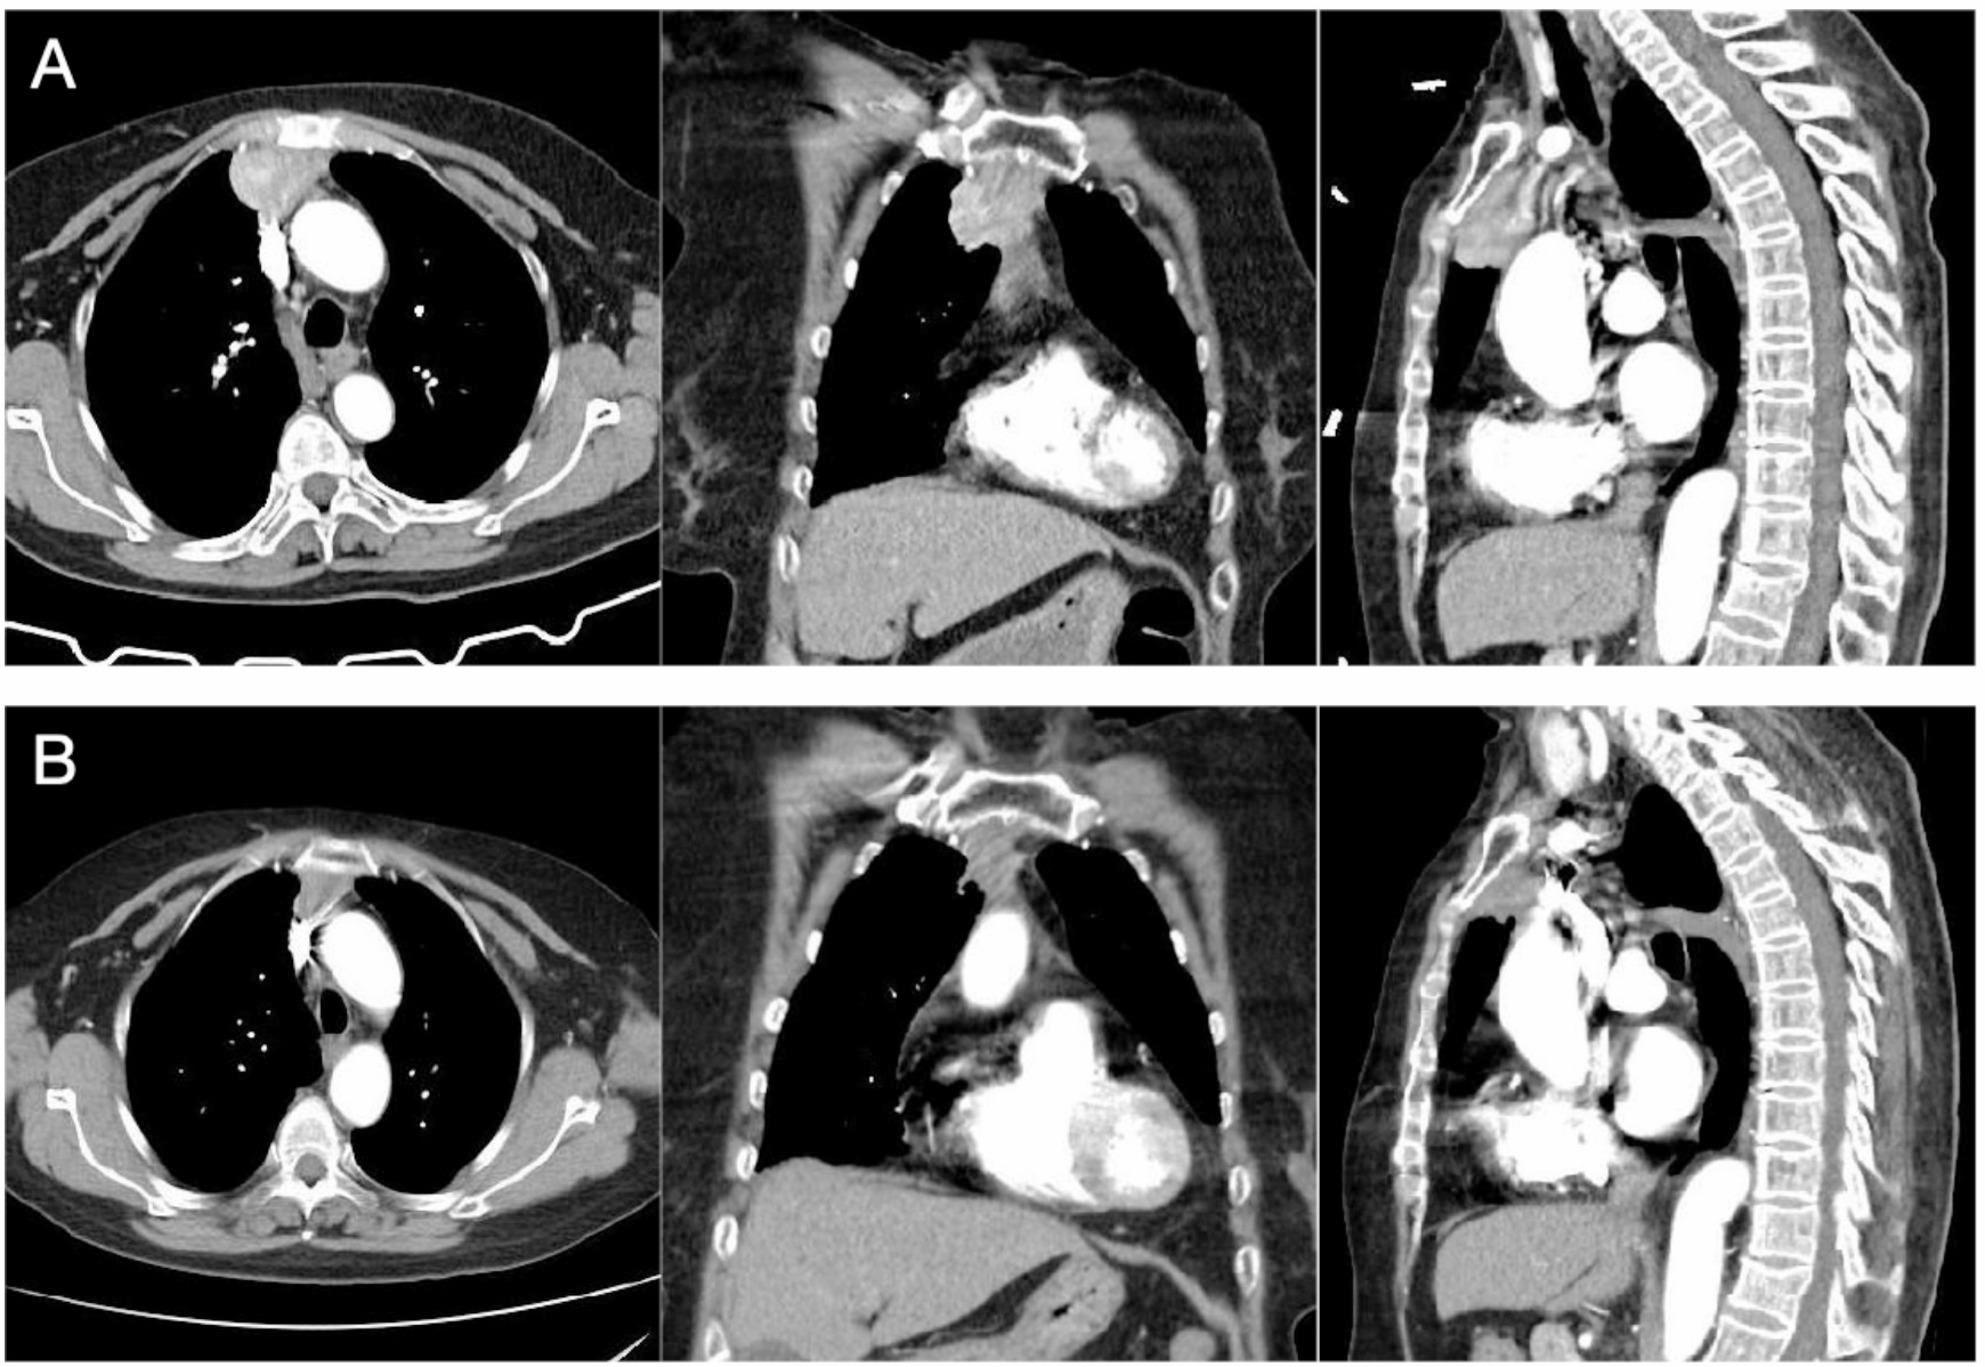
Pre- and post-neoadjuvant CT of thymic carcinoma invading the SVC. A Pre-treatment contrast-enhanced CT showing a lobulated anterior mediastinal mass invading the SVC and right upper lobe; B Post-neoadjuvant CT demonstrating partial response with tumor shrinkage
